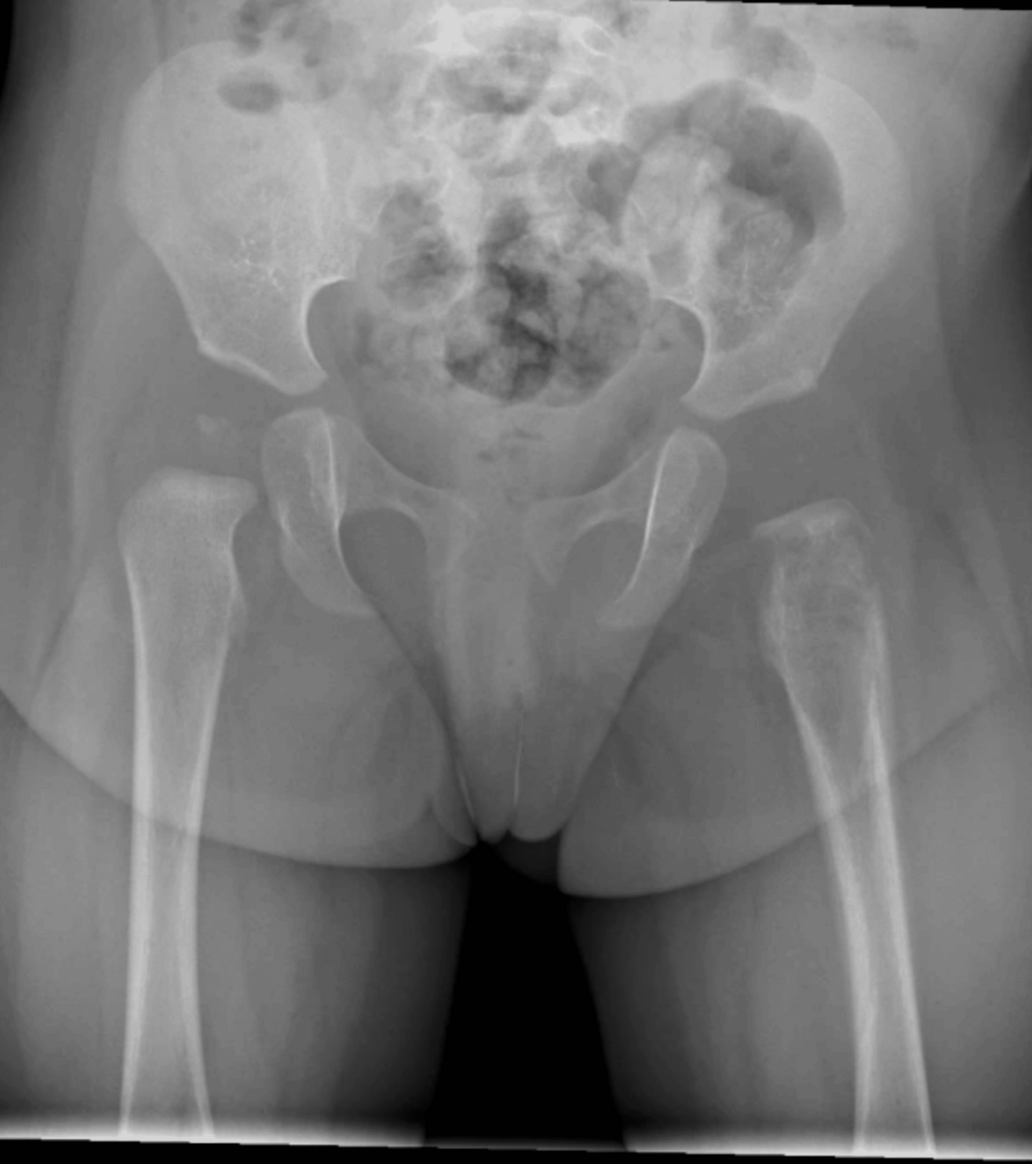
X-ray of the pelvis including the midshaft of both femurs X-ray showing an osteolytic lesion in the proximal metaphysis of the left femur with indistinct margins, cortical destruction, and a layered periosteal reaction along the femoral shaft, suggestive of an aggressive bone process.
